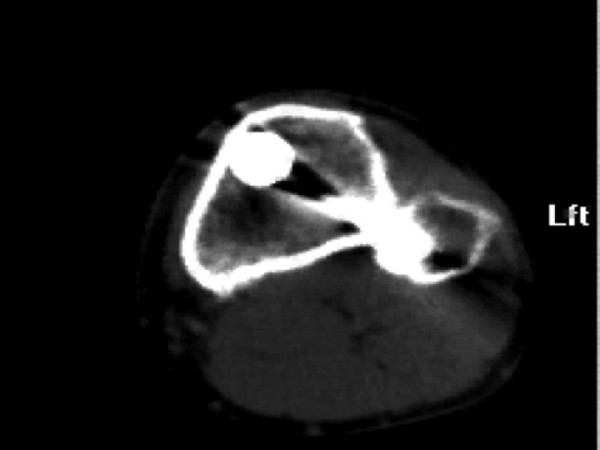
Computed tomography in axial cut showing the screw crossing the proximal tibiofibular joint.
